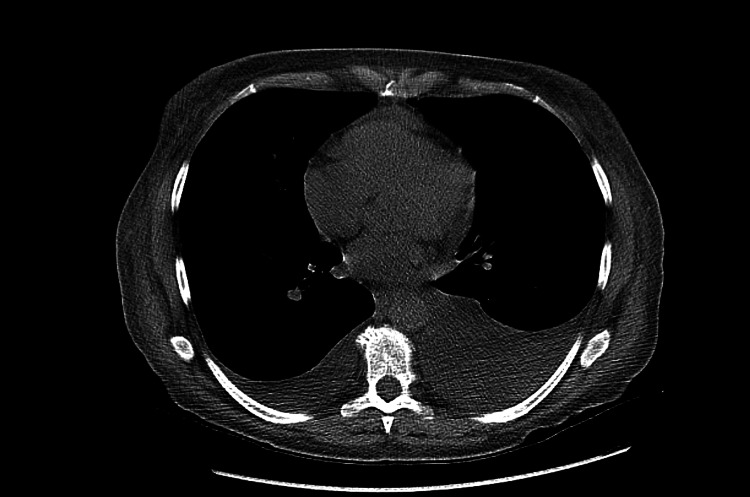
CT abdomen and pelvis with signs of pleural effusion
